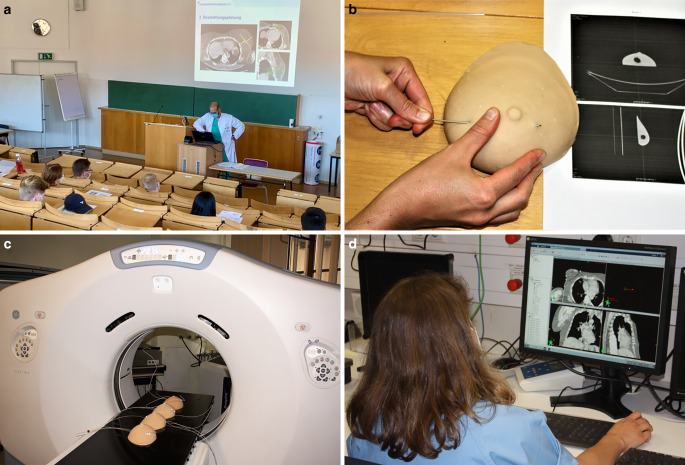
Training course on breast cancer brachytherapy using the newly developed breast cancer model. Following an introductory lecture (a), residents and participants trained the implantation of single-lead catheters into the silicone breast model (b). CT scan evaluated the accuracy of applicator placement and CT-based contouring and treatment planning was demonstrated and trained (c, d)
